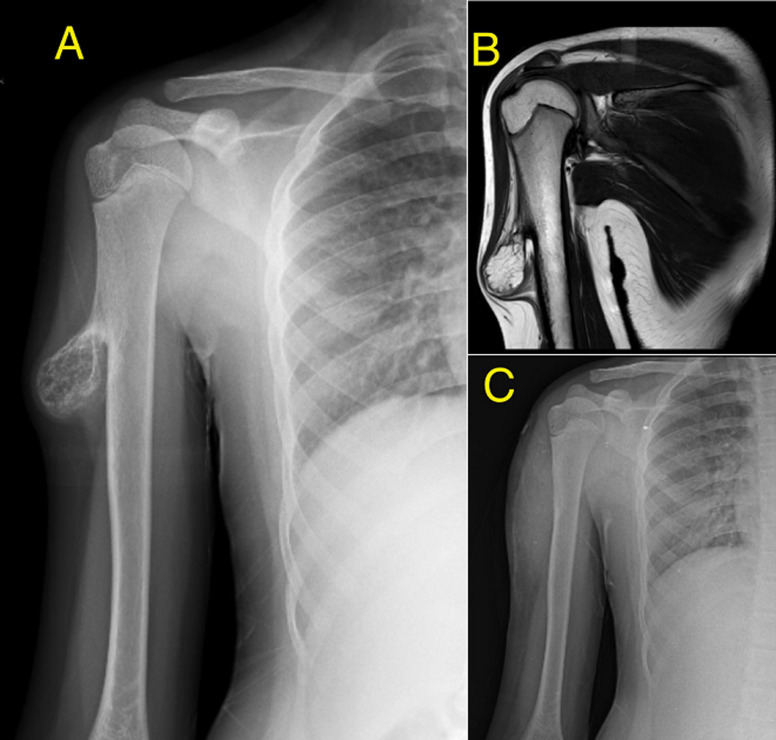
A) right humerus showing irregular outgrowth; B) T2-weighted MRI of right humerus showing bony outgrowth arising from the lateral aspect of diaphysis; C) postoperative radiography showing cosmetic improvement
